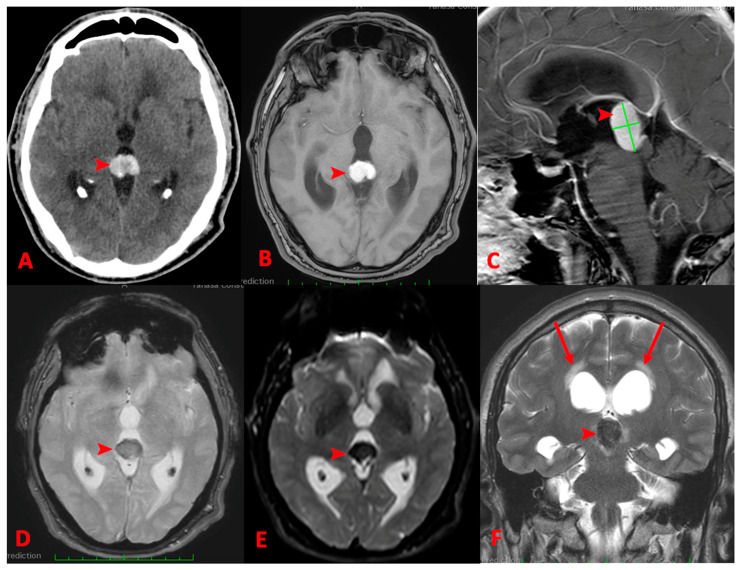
Preoperative images (A) nonenhanced computer tomography showing hyperdense mass at the level of pineal gland. The lesions are hyperintense in T1 (B,C), hypointense in T2 (D), and exhibit marked reduced diffusion on DWI (E). The lesion is compressing the tectal plate, obstructing the cerebral aqueduct and determining active hydrocephalus (arrows) (F).
